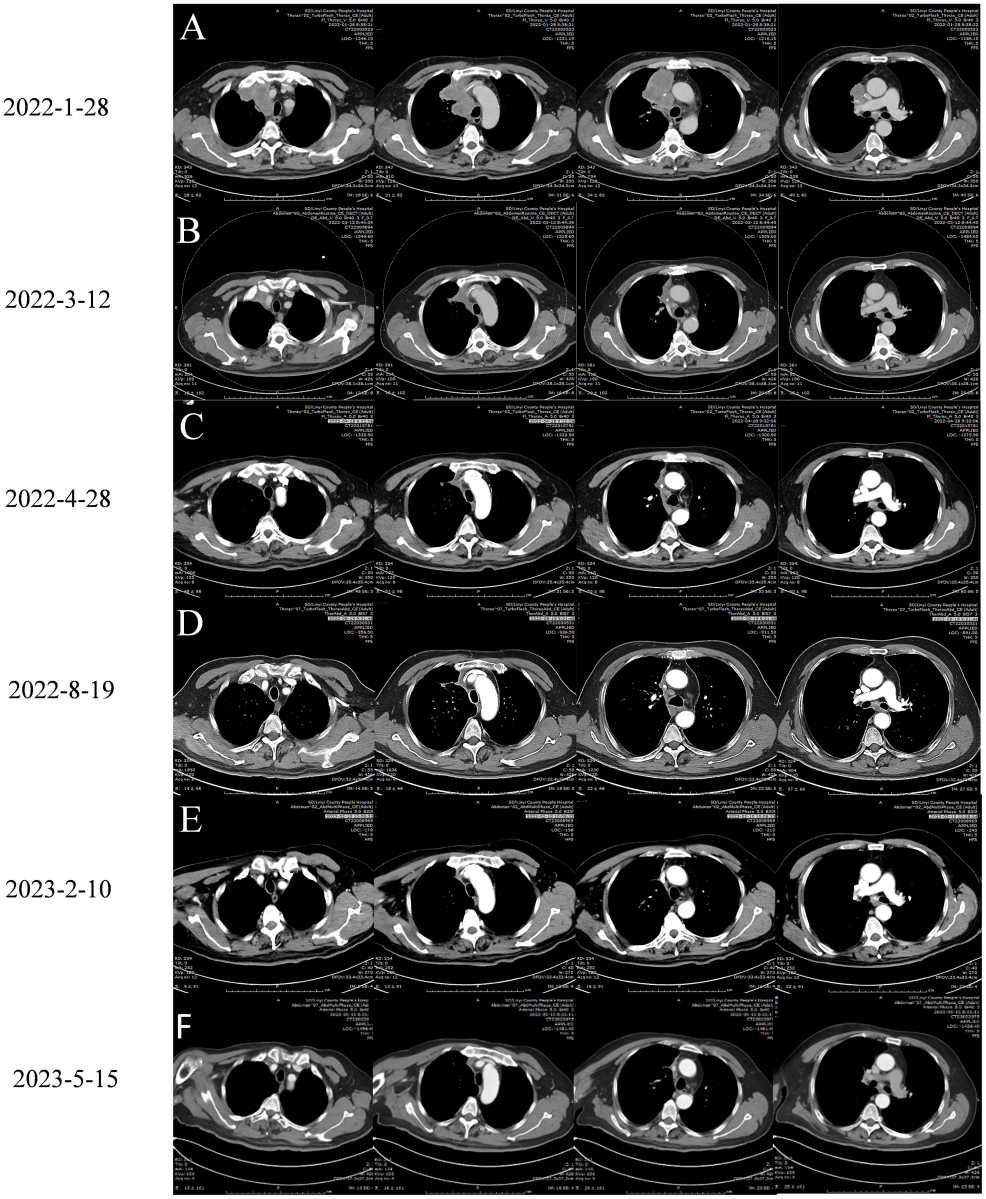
Chest enhanced CT images of the patient before and after treatment with Tislelizumab Combined with Anlotinib:arterial phase imaging. (A) January 28, 2022 is the chest CT image before treatment. (B) On March 12, 2022, chest CT images of two cycles of treatment with Tislelizumab Combined with Anlotinib. (C) On April 28, 2022, chest CT images were obtained after 4 cycles of treatment with Tislelizumab Combined with Anlotinib. (D) Chest CT images of 9 cycles of treatment with Tislelizumab Combined with Anlotinib on August 19, 2022. (E) On February 10, 2023, a chest CT image was obtained. The patient underwent a total of 13 cycles of treatment with trastuzumab and anlotinib, as well as 2 doses of trastuzumab monotherapy. (F) Chest enhanced CT scan on May 10, 2023, patient has terminated treatment for 3 months.
